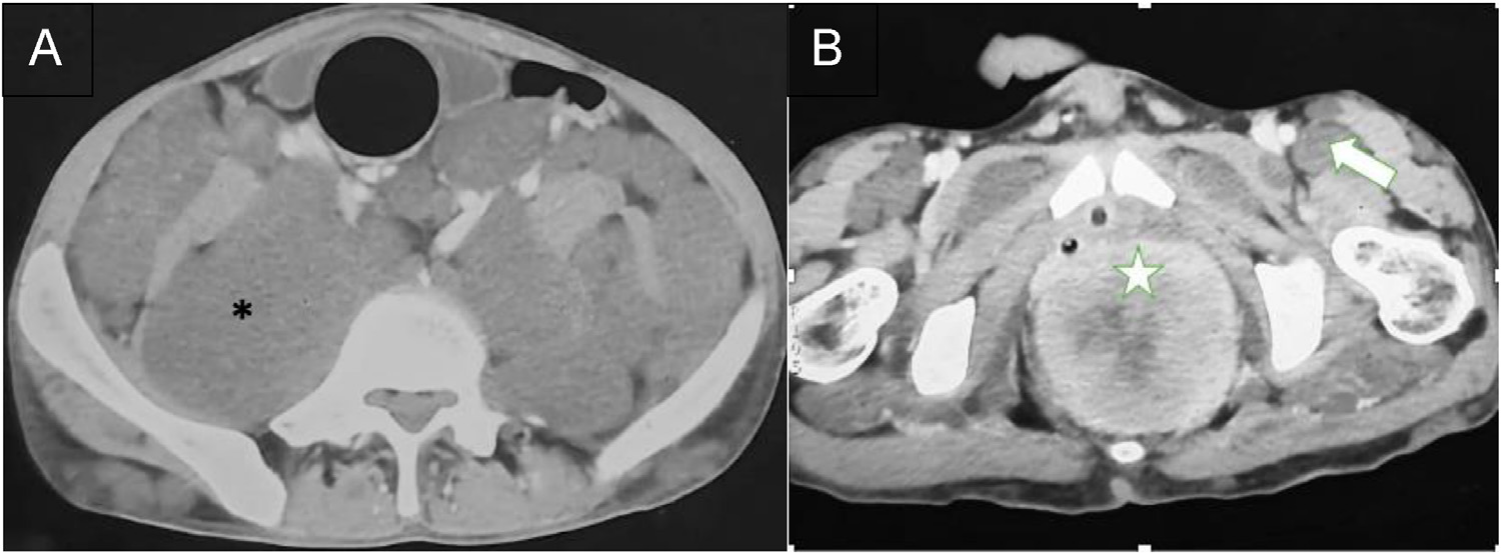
Contrast-enhanced axial section of abdominal CT scan (A,B) depicted a large heterogenous mass (B, white star) that seemed to arise from the prostate and several numerous small low attenuation masses in intra-extraperitoneal (A, asterisk) and also along the inter-intramuscular (B, white arrow).
